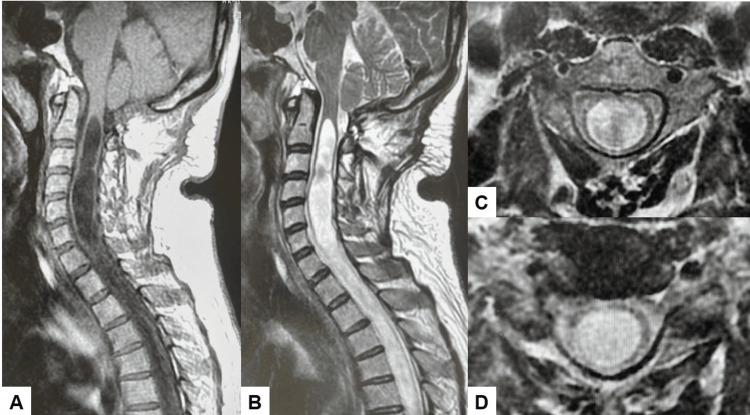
MRI after FMD: (A) T1 sagittal MRI, (B) T2 sagittal MRI, (C) T2 axial MRI at C4, and (D) T2 axial MRI at C5/6. The large syrinx was a little decreased. MRI: magnetic resonance imaging; FMD: foramen magnum decompression
